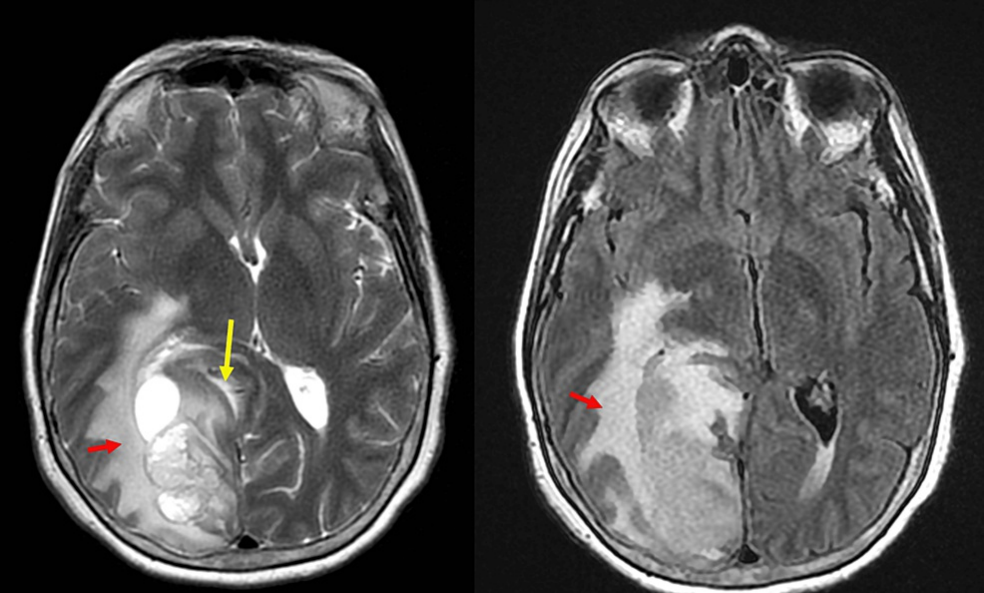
Initial axial T2 propeller (Left) and axial T2 flair (Right) MRI of the head showing a large T2 hyperintensity with poorly defined edema [red arrow], with mass effect in the right posterior parietal and occipital lobes. This corresponds to the area of vasogenic edema on the CT (Figure 1). There is also compression of the posterior horn of the right lateral ventricle [yellow arrow].
